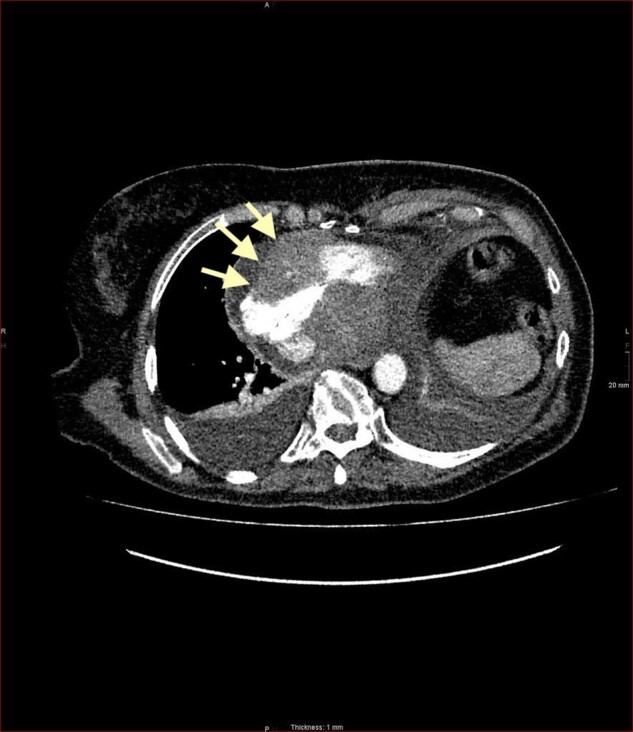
Lobulated soft tissue mass extending into the musculature of the right atrium and bilateral pleural effusions on computed tomography thorax.
